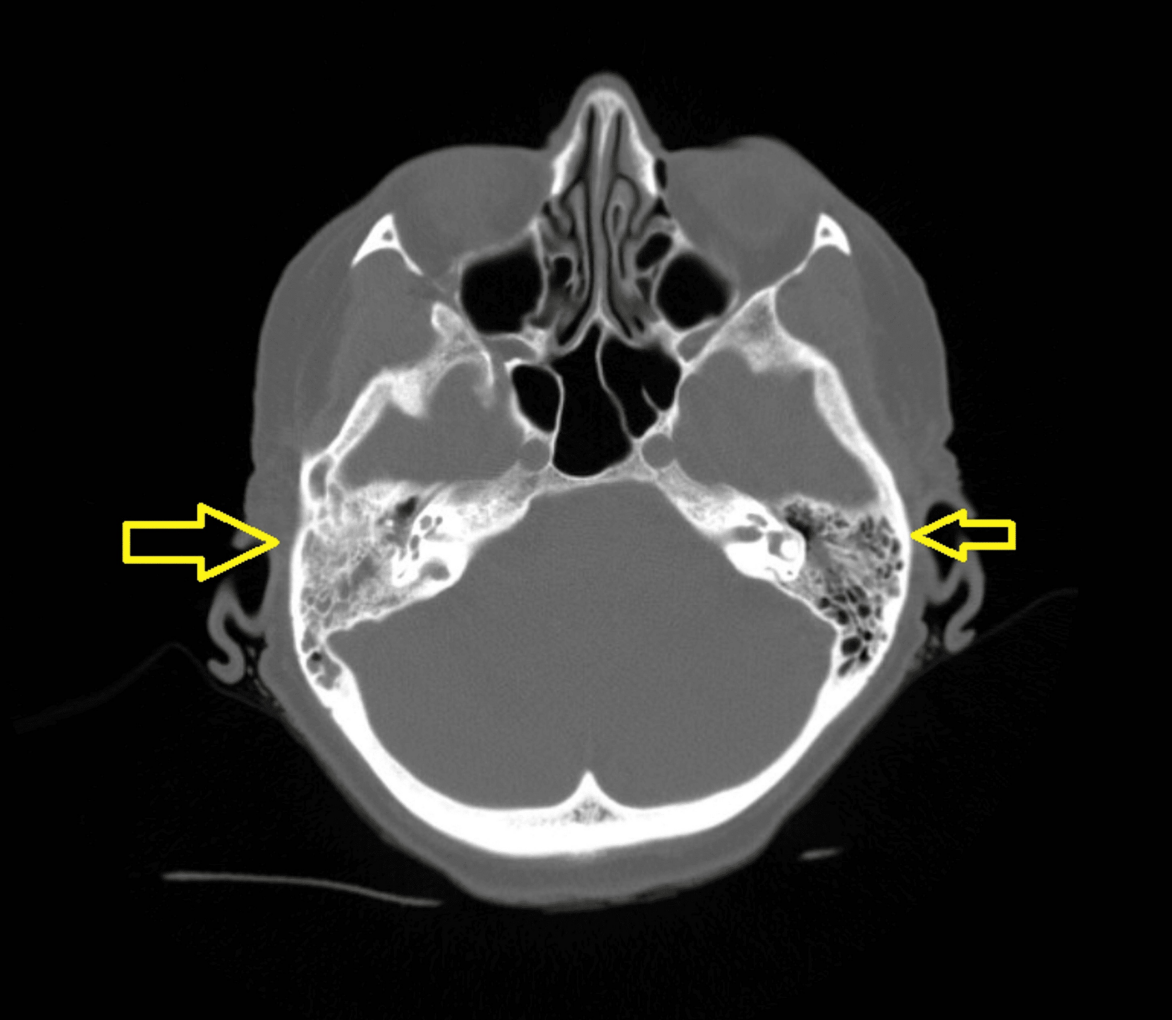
Findings from the patient’s computed tomography (CT) of the head indicating otomastoiditis, with a right-sided predominance as indicated by the larger arrow.
